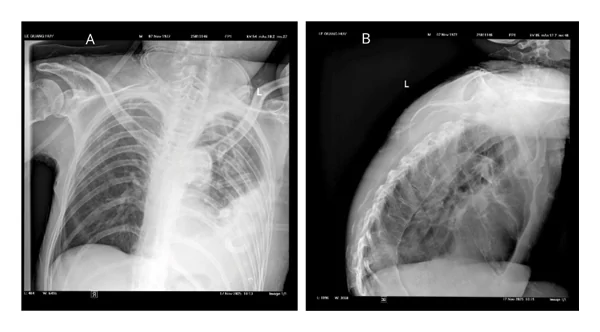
Anteroposterior (A) and lateral (B) radiographs of the cervical and thoracic spine demonstrating a fixed kyphotic cervicothoracic deformity secondary to ankylosing spondylitis.
